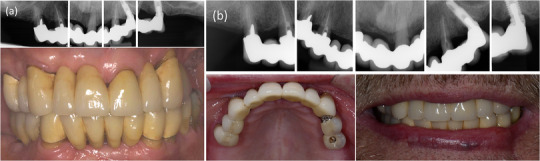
(a) Radiographs and clinical photos of patient‐ID‐105 after 13 years of implant function. (b) Radiographs and clinical photos of the same patient after 20 years of implant function.
